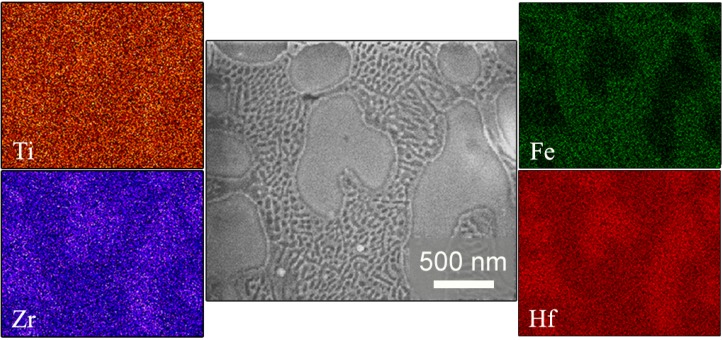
SEM image of a central region of the Ti_40_Zr_20_Hf_20_Fe_20_ sample, together with the compositional energy dispersive X-ray mappings corresponding to Ti, Zr, Fe, and Hf elements.
